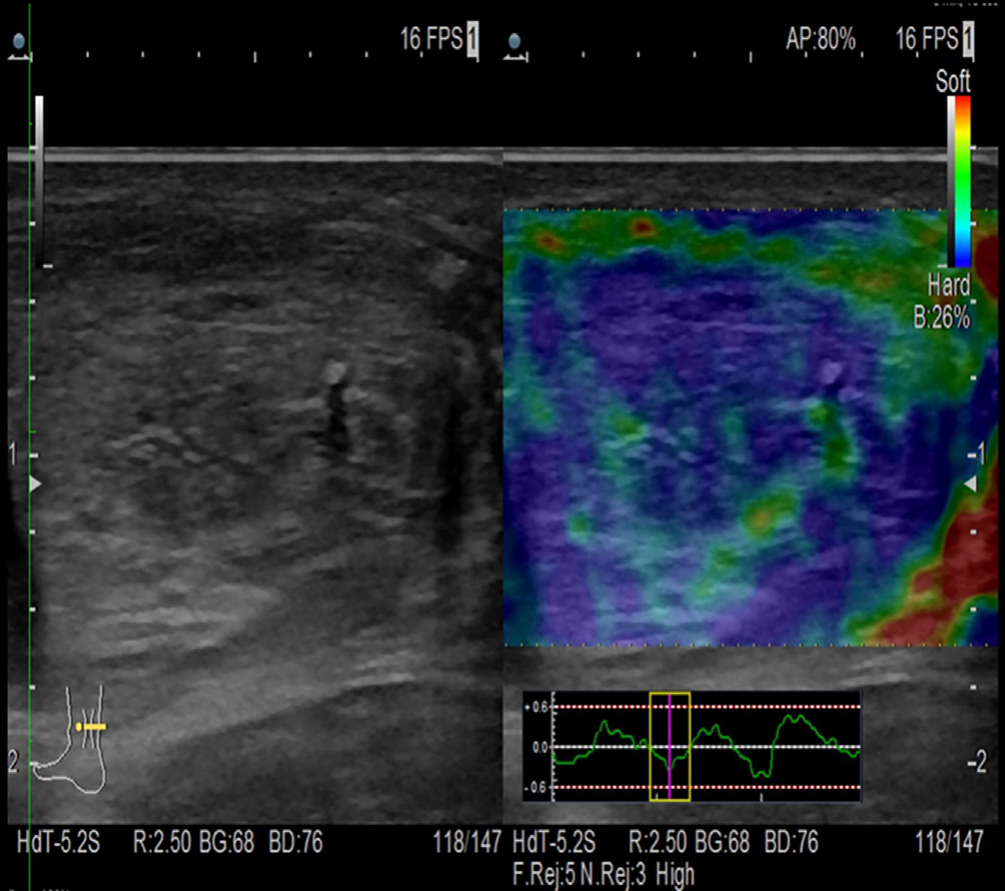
In the left part of the image: B‐Mode ultrasound study. In the dx part of the image: elastosonographic study with good representation of blue areas demonstrating recovered tendon stiffness.
